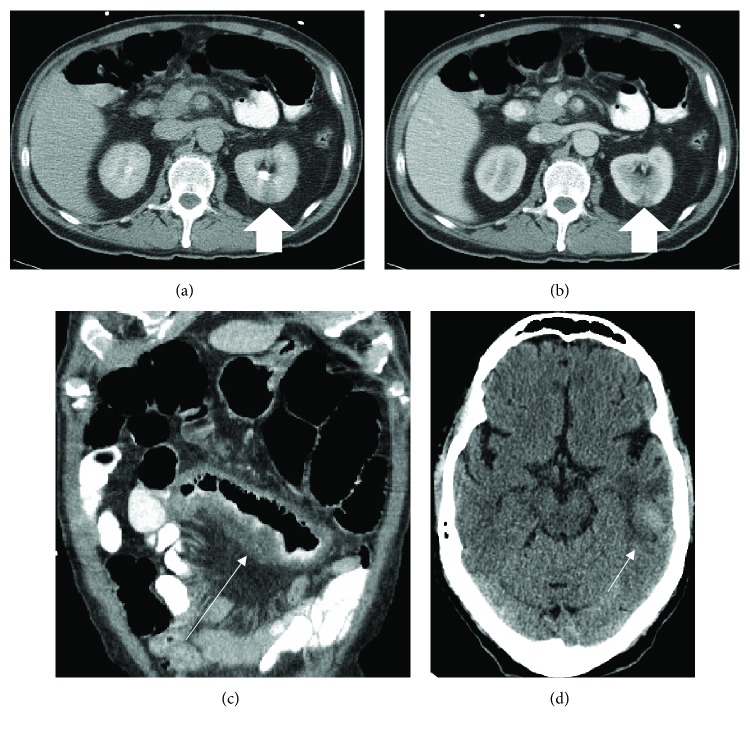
(a, b) Axial contrast-enhanced abdominal CT demonstrates a segmental infarct in the posterior aspect of the left kidney (short thick arrow). (c) Coronal reformatted CT showing infarcted small bowel (long thin arrow). (d) Noncontrast head CT showing a subacute hemorrhagic infarct in the left temporal lobe (short thin arrow).
